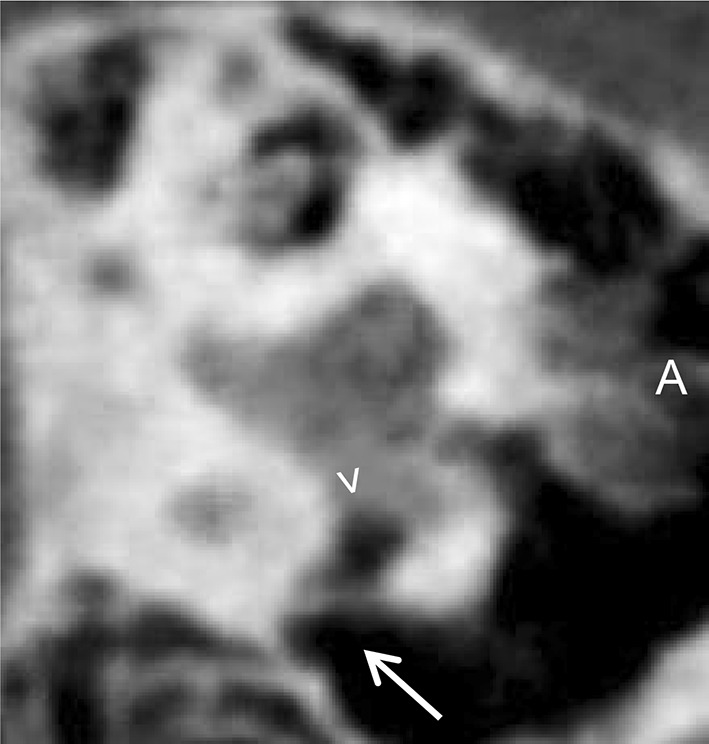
Sagittal view of a left temporal bone in CBCT imaging. The RWN ( >) can be clearly identified but seems capped by a secondary membrane ( →)
